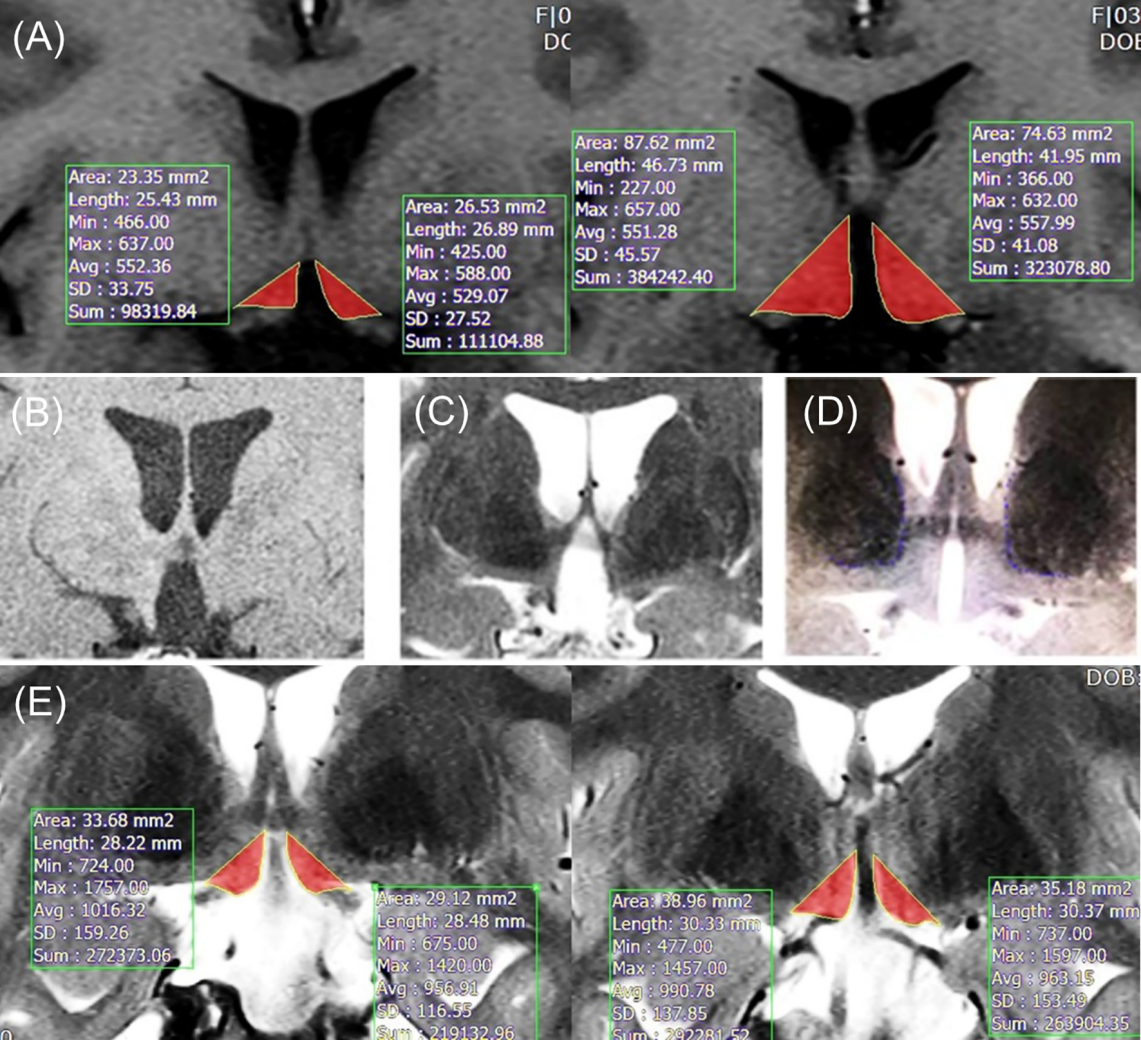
(A) Example of method 1 in T1-weighted images. (B) T1-weighted image with severely damaged HT (optic tract displacement is not observed). (C) T2-weighted image with severely damaged HT (optic tract displacement is observed). (D) T2-weighted image with normal HT. (E) Example of individually tailored method in T2-weighted images. Lateral borderline (the edge of optic tract) was adjusted for each patient’s thalamus borderline. HT, hypothalamus.
